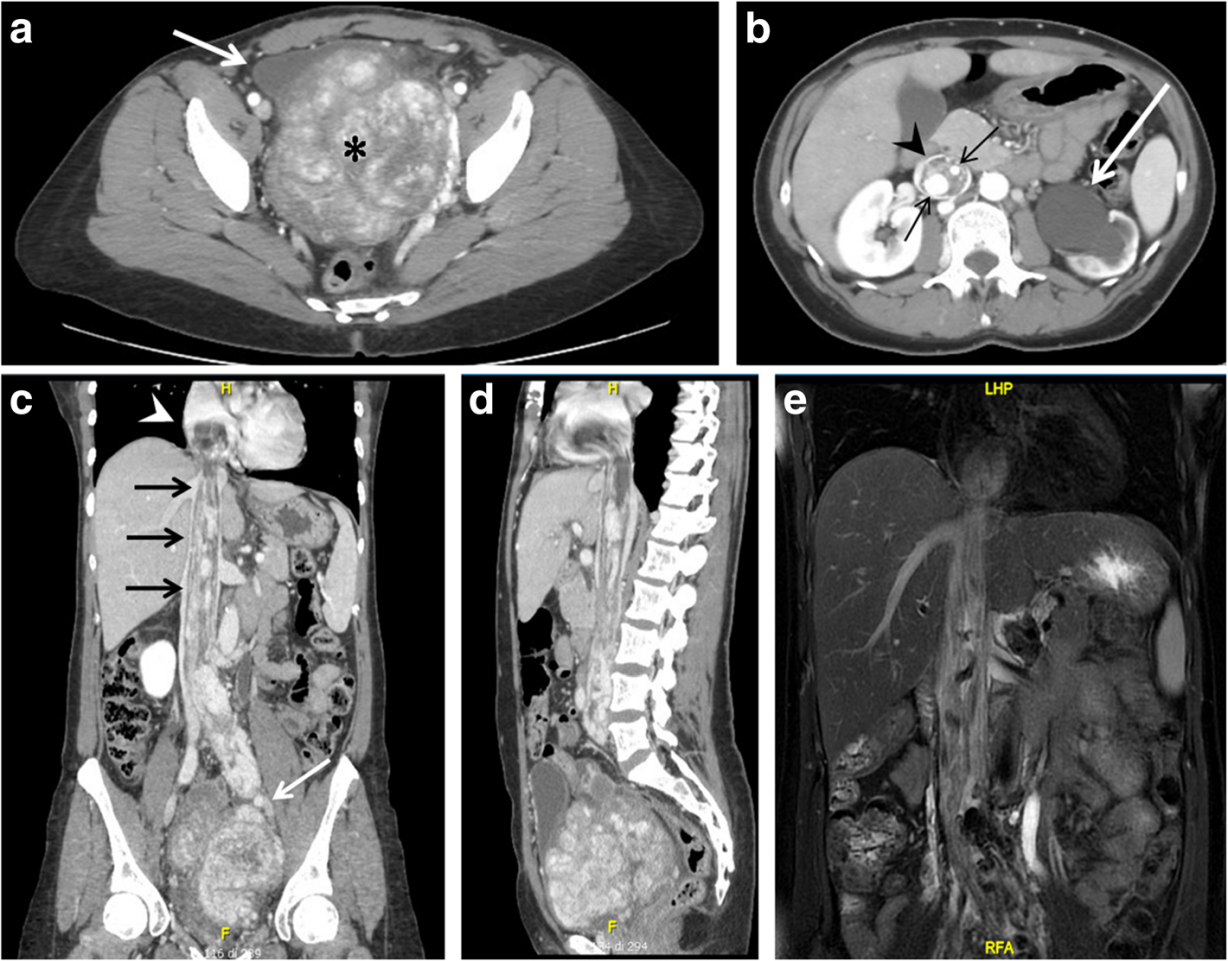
Chest – abdomen – pelvis CT scan (a-d) and MR (e). a. Axial projection showing a voluminous pelvic mass of 15 × 22 × 9 cm (*asterisk*), with significant contrast enhancement due to hypervascularization, causing *right* antero-lateral dislocation of the bladder (*arrow*). b. The mass also determines left hydro-ureteronephrosis (*white arrow*). Notice the presence of contrast-enhanced large vessels (*black arrows*) within the IVC (*arrowhead*). c-d. Coronal and sagittal CT projections showing the pelvic tumor directly extending into the left iliac vein (*white arrow*), IVC (*black arrows*) and occupying the right atrium (*arrowhead*). e. Coronal MR projection showing the caval and cardiac extension of the tumor
